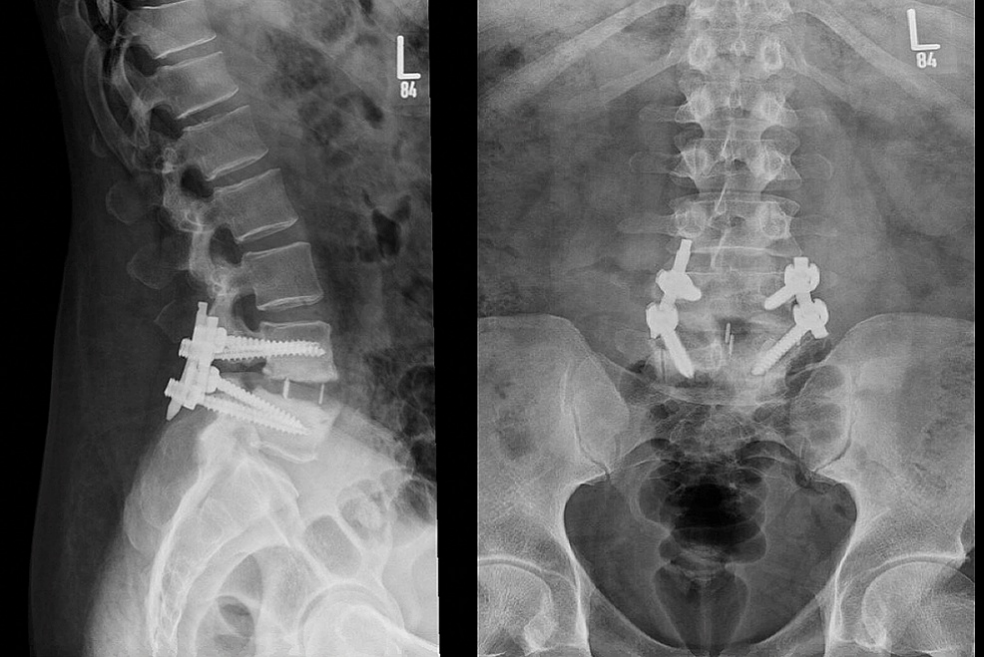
Lateral and AP X-rays of lumbar spine one-month post-revision.
